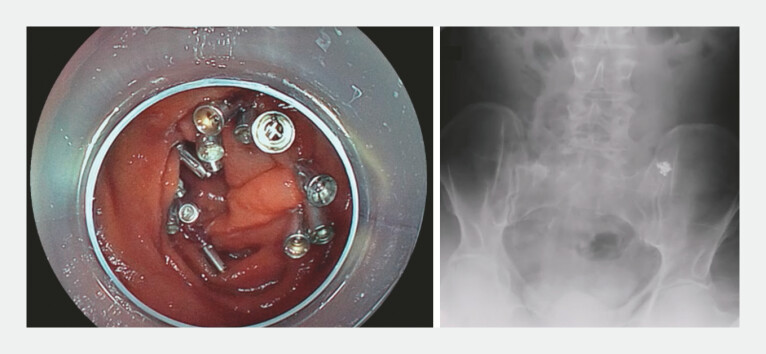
A white light image and an abdominal radiography after complete suturing of the mucosal defect. The mucosal defect was completely closed by the origami method using endoscopic clips (SureClip; Boston Scientific Japan, Tokyo, Japan). The radiographic image indicates that the treated lesion was identified in the pelvis, and the procedure was completed without perforation.
